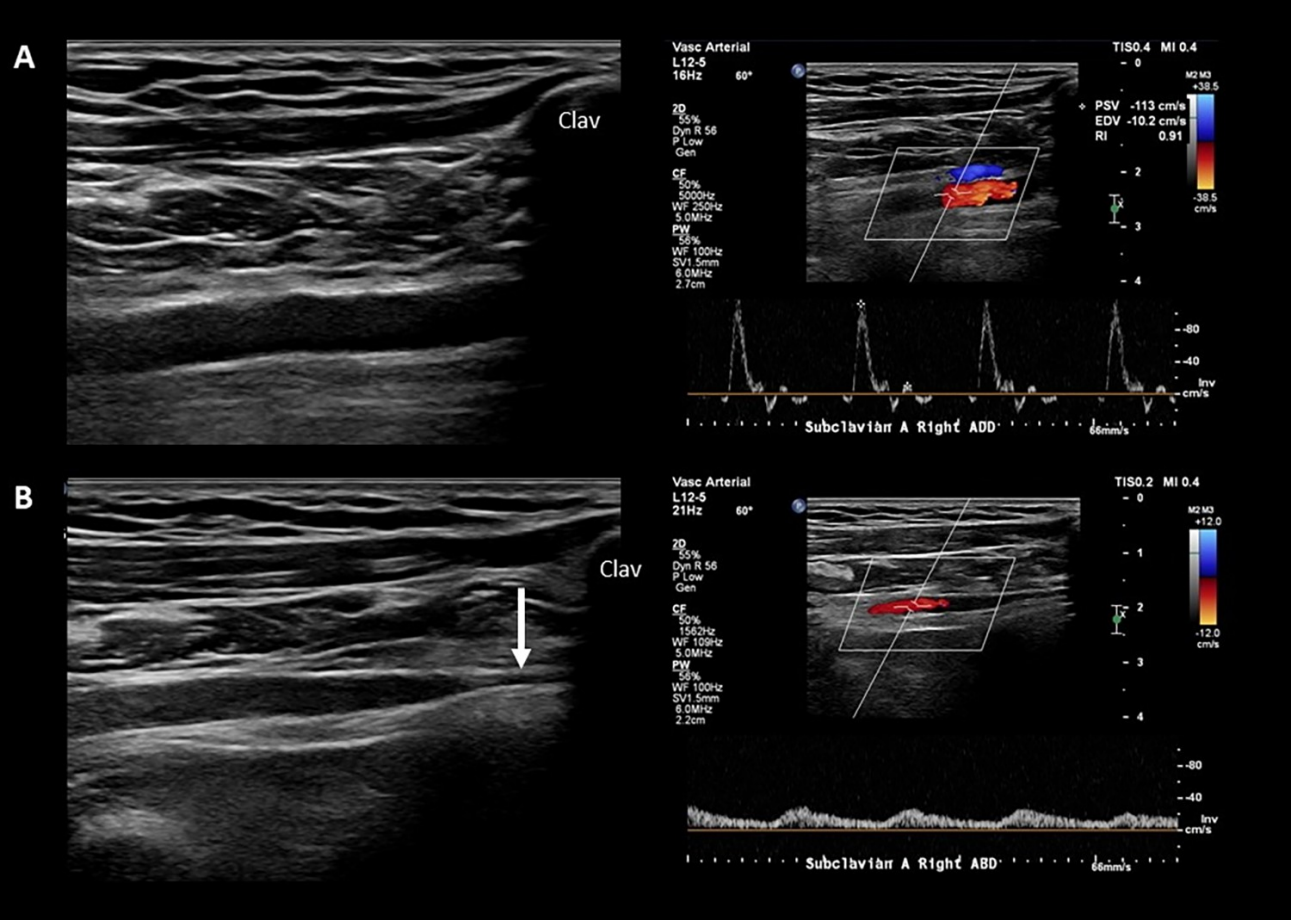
Arterial measurements in TOS. Vascular ultrasound (B mode and PW mode) of the right subclavian artery of a patient with arterial (supra- + costoclavicular) TOS. A shows the artery in the immediate infraclavicular region in neutral arm position, and B during arm abduction. In the neutral position, normal triphasic flow is seen. Note that during arm abduction the artery becomes severely narrowed (arrow, ‘funnel sign’) as it leaves the costoclavicular space, associated with a tardus-parvus type postocclusion blood flow, loss of pulse, and severe diffuse pain and numbness of the arm. Clav: clavicula.
